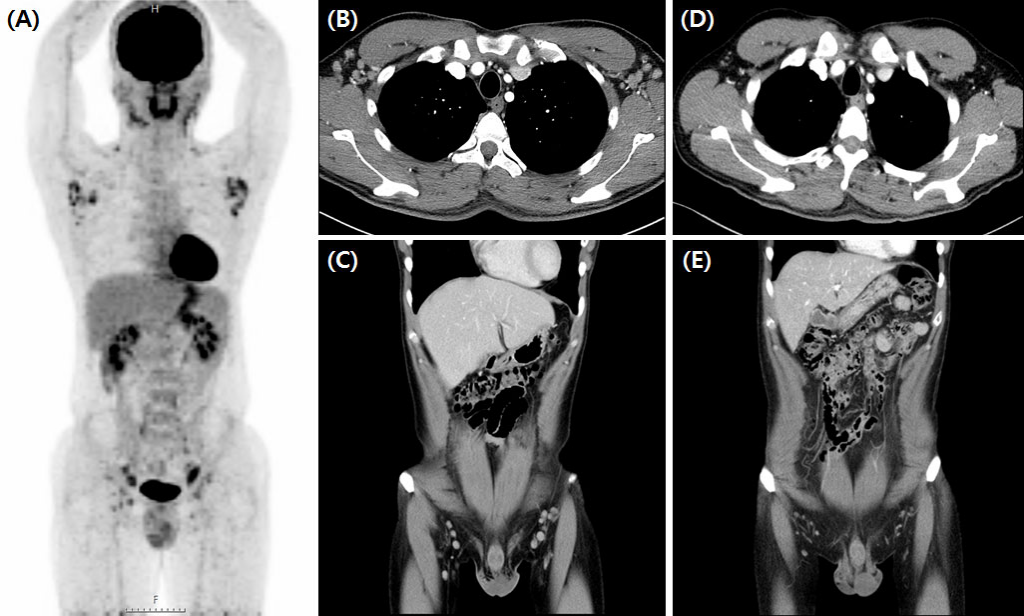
Significant regression of enlarged lymph nodes after third siltuximab infusion. Initial FDG-PET CT scan of iMCD plasma cell type patient (A) showed MCD involving bilateral cervical, supraclavicular, axillae, common and external iliac, and inguinal lymph nodes. CT scan findings before (B, C) and after (D, E) siltuximab infusion showed markedly regressed MCD-involved lymph nodes in the bilateral inguinal and axillar areas. FDG-PET CT, 18-fluoro-2-deoxy-D-glucose positron emission tomography-computed tomography; iMCD, idiopathic multicentric Castleman disease.
